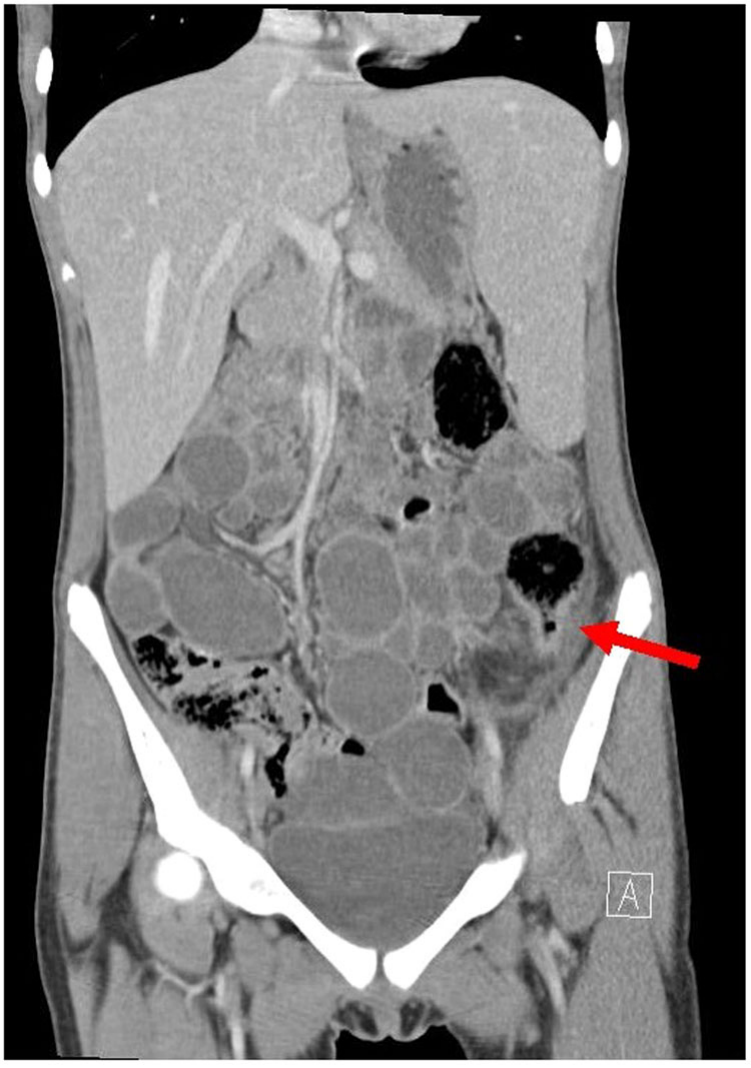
Computed tomography abdomen and pelvis with IV contrast. Coronal image showing abnormal narrowing at the descending colon as denoted by the arrow.
